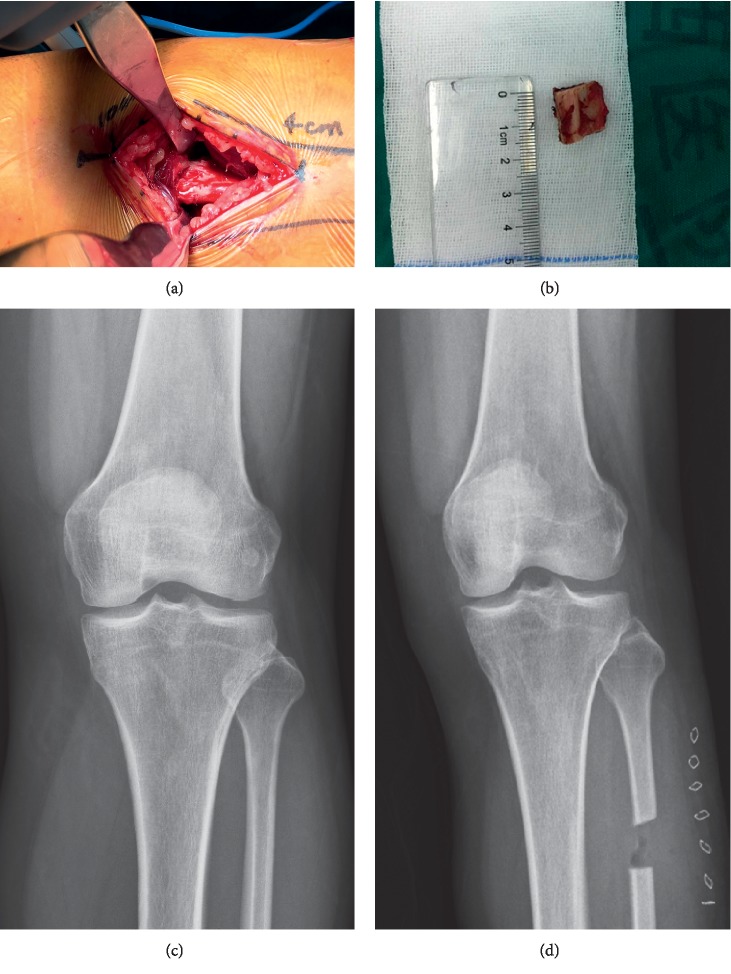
(a) Intraoperative clinical image showing an incision carried out along the fibula between 4 and 10 cm from the fibular head. The upper section of the fibula was exposed from the intermuscular space by tissue separation. (b) An osteotomy of 1 cm was performed between 4 and 6 cm from the proximal fibular head. (c, d) Knee radiograph before and after upper partial fibulectomy.
